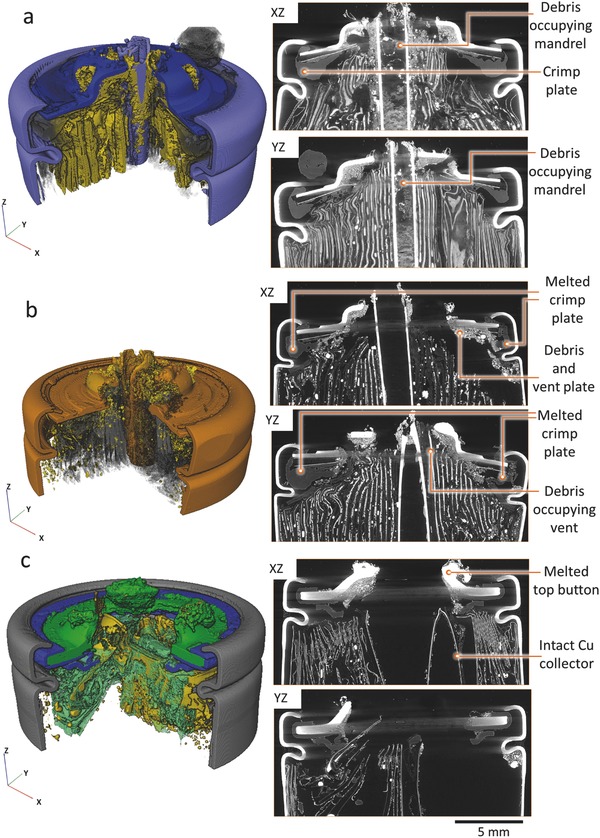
Postmortem 3D reconstructions and corresponding *XZ* and *YZ* orthoslices of a) Sanyo, b) Panasonic, and c) Samsung cells showing the damage to the top button after thermal runaway. The cylindrical mandrel in (a) and (b) is seen to protrude after puncturing the top button. The radiography movies showing the process of thermal runaway for the Sanyo, Panasonic, and Samsung cells are provided as Movies S5, S7, and S8 in the Supporting Information.
